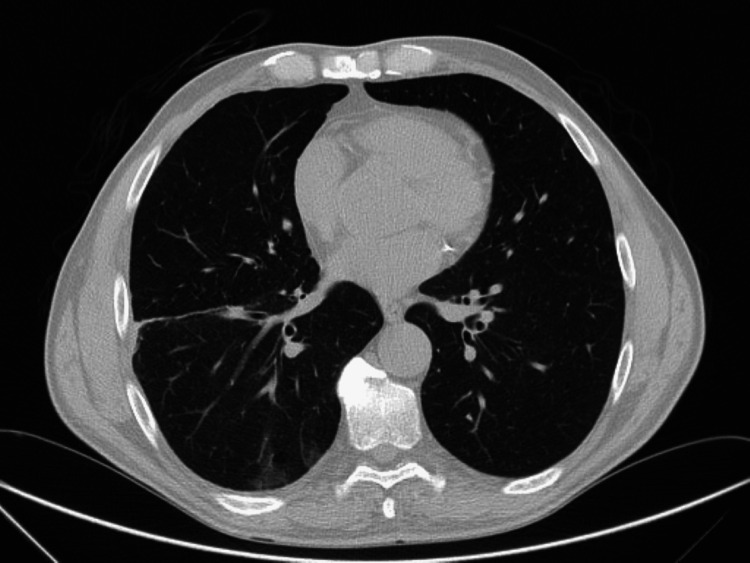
Thoracoabdominal CT scan performed five months after diagnosis, with marked improvement after antibiotic therapy (axial view).
